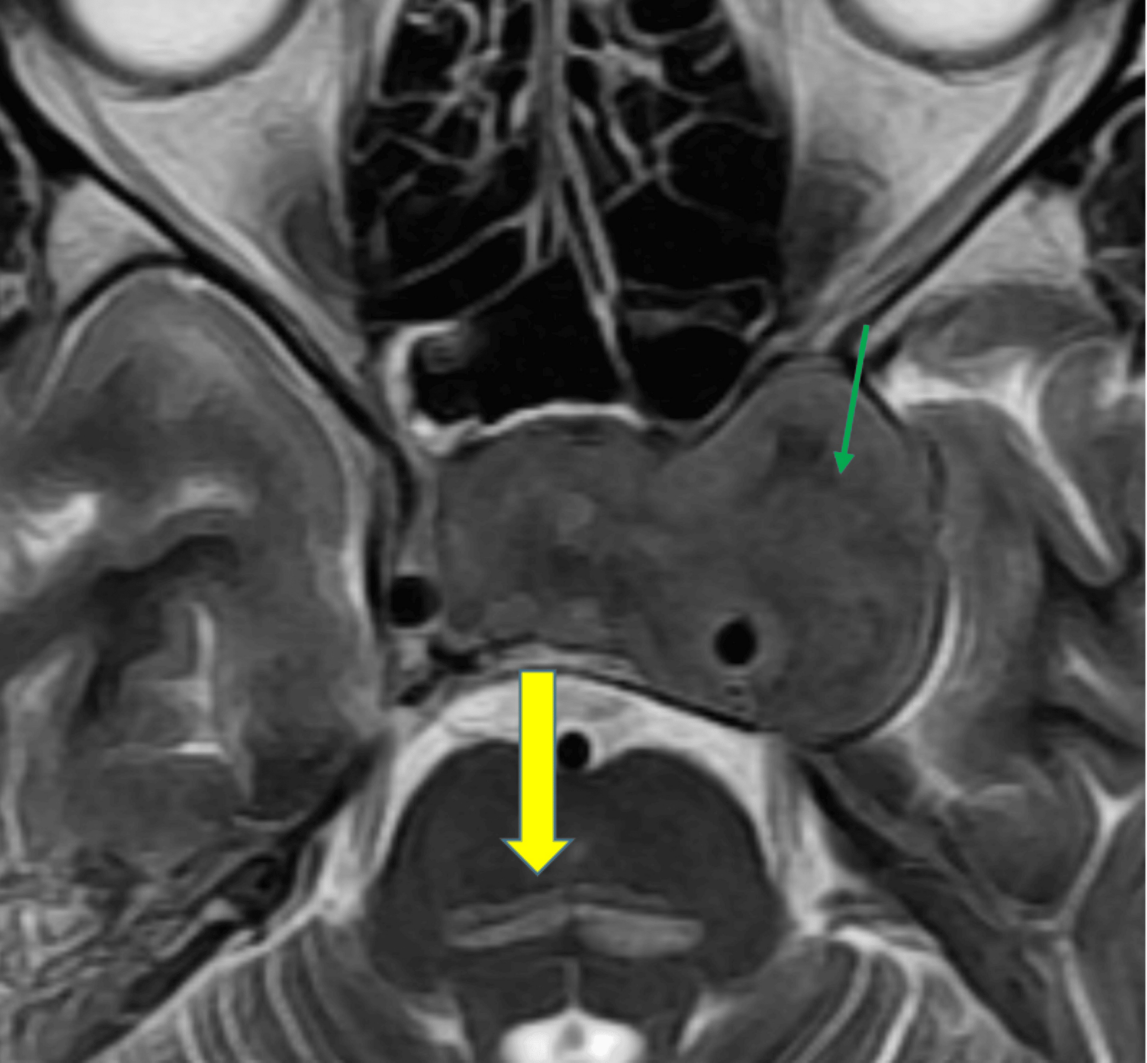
T2 Axial MRI Sequence Showing Pontine Myelinolysis (Yellow Arrow) and a Large Pituitary Macroadenoma (Green Arrow). MRI: magnetic resonance imaging
